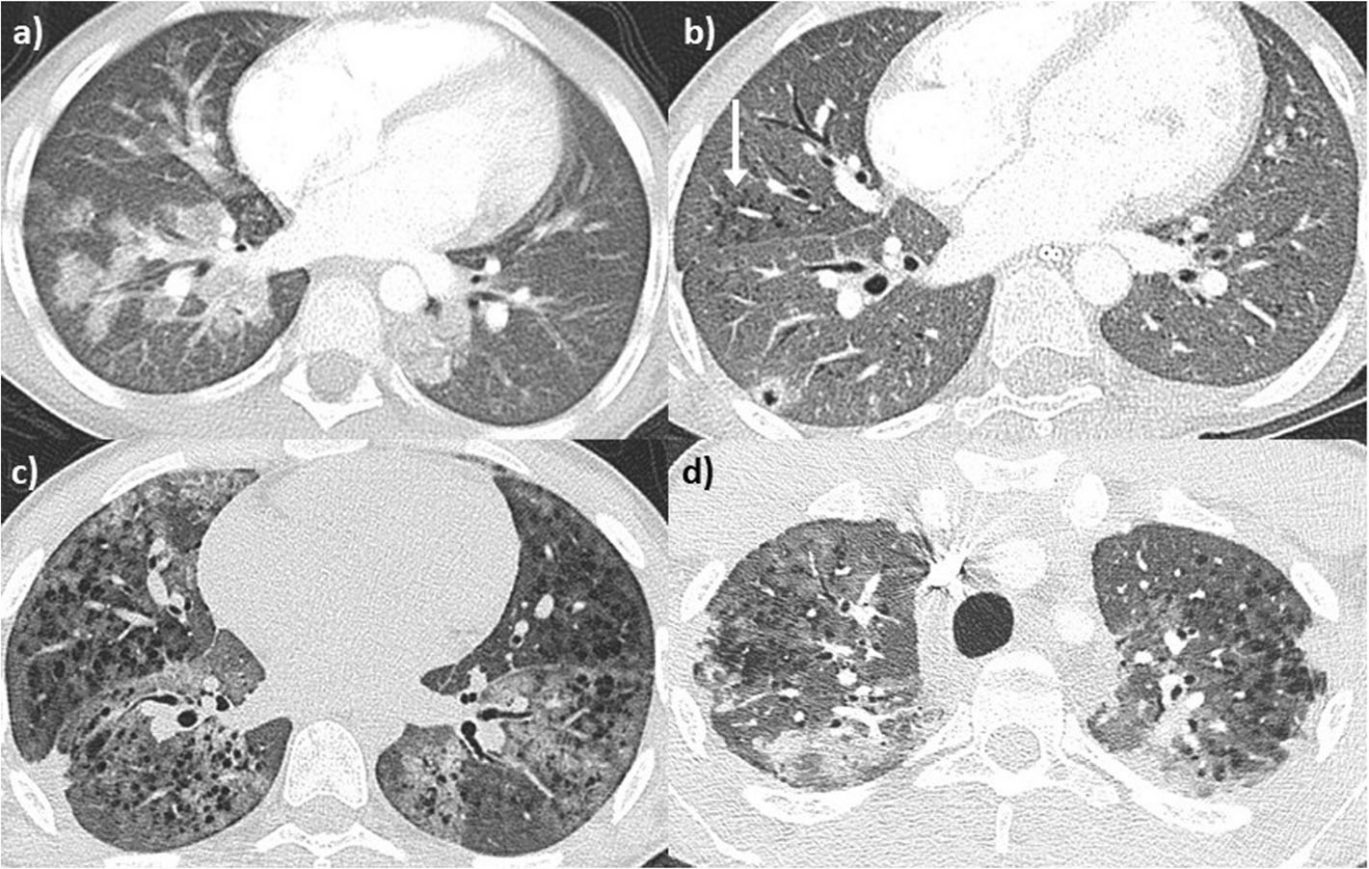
Patient 3. Radiologic evolution. Axial CT images of the lungs; a: At 9 years old the patient presented with an acute flare, post-contrast CT demonstrates ground glass nodules which resolved on follow up chest radiographs (not shown); b: At 11 years old a cavitating pulmonary nodule is present in the right lower lobe, and emphysema has developed surrounding the pulmonary vessels (arrow). The pulmonary nodule resolved on interval CT (not shown); c: At 15 years old the emphysema has proliferated, with a perivascular component again present. An acute ground glass infiltrate is also present; d: At 20 years old, peripheral polygonal areas of consolidation are present within the peripheral pulmonary parenchyma/pleura, in keeping with pleuroparenchymal fibroelastosis. A ground glass infiltrate is also present, as is emphysema
